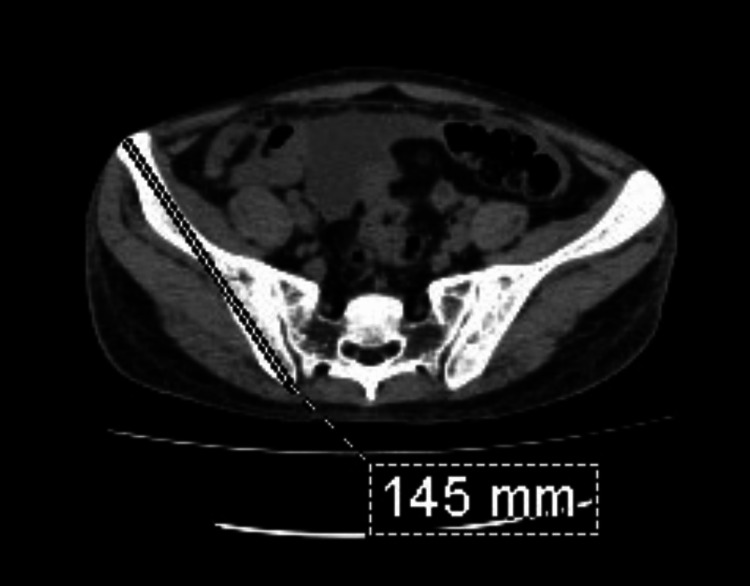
Axial CT section of the pelvis at the level of sacral ala. The line joins the anterior-superior iliac spine and the sacroiliac joint on the right side.
